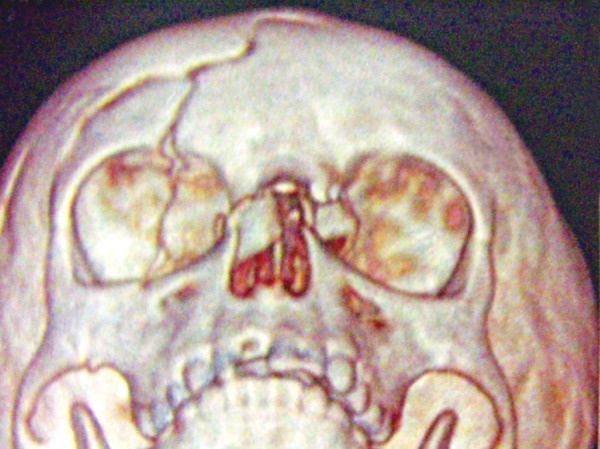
3D CT scan shows fractured right frontal bone and fractured nasal bone
